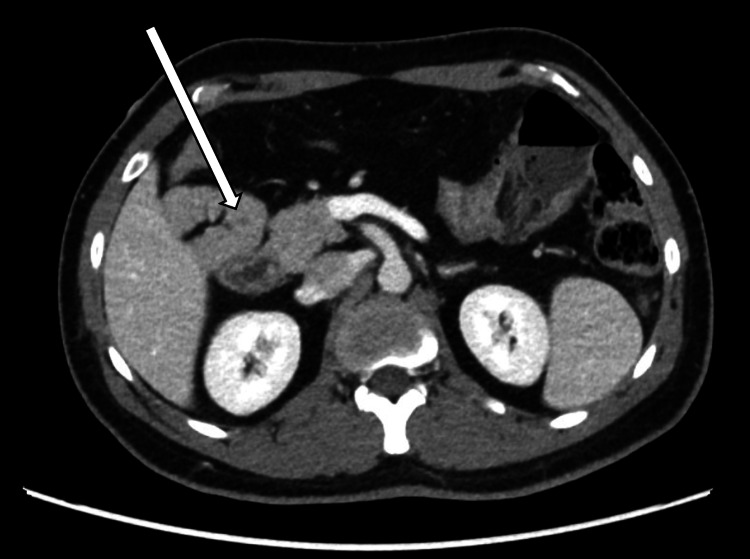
Failure of the duodenojejunal junction to cross the midline Axial CT image demonstrating the duodenojejunal flexure, which fails to cross the midline and is located on the right side of the abdomen, consistent with intestinal malrotation. CT, computed tomography.
